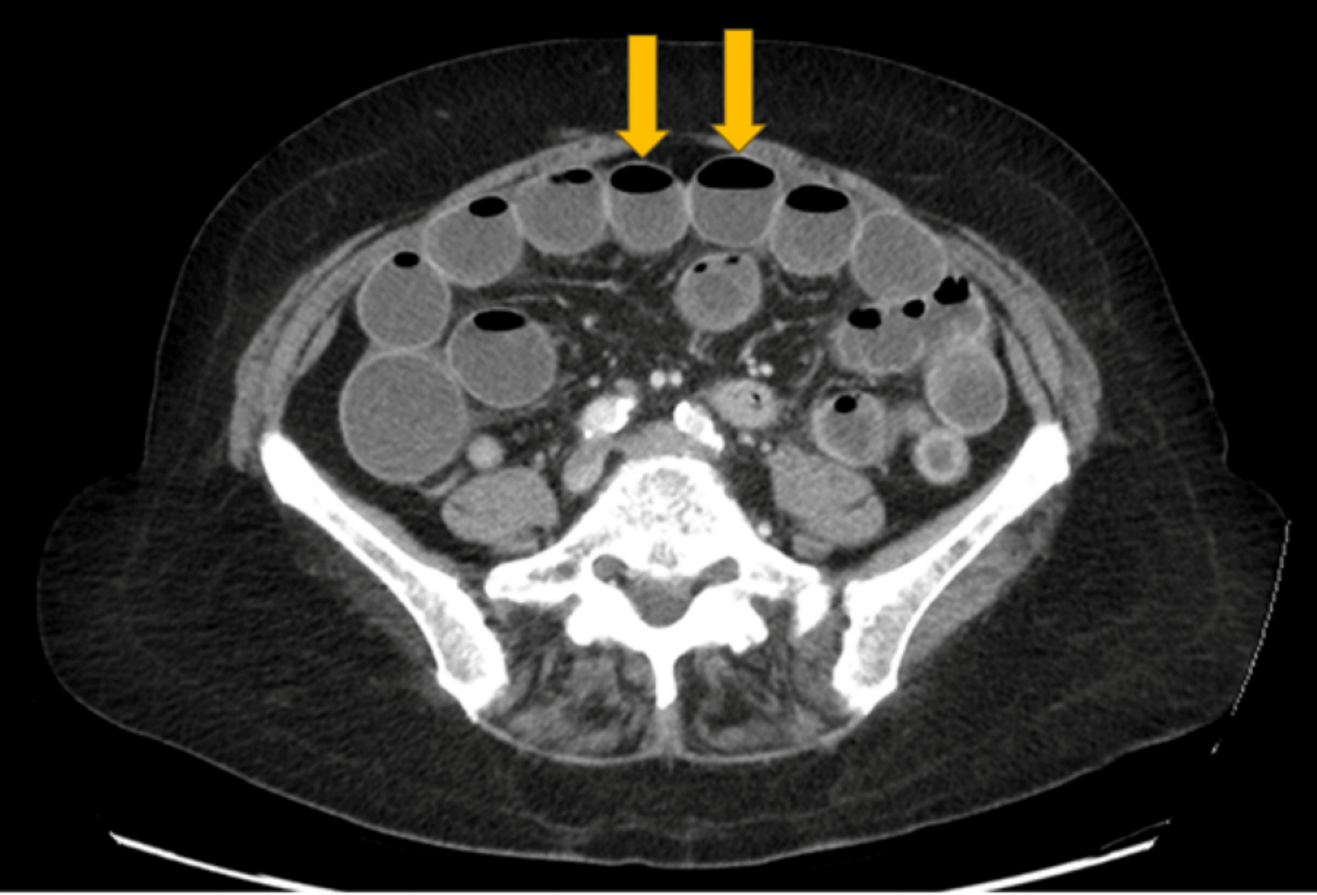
Example of small bowel obstruction on CT
